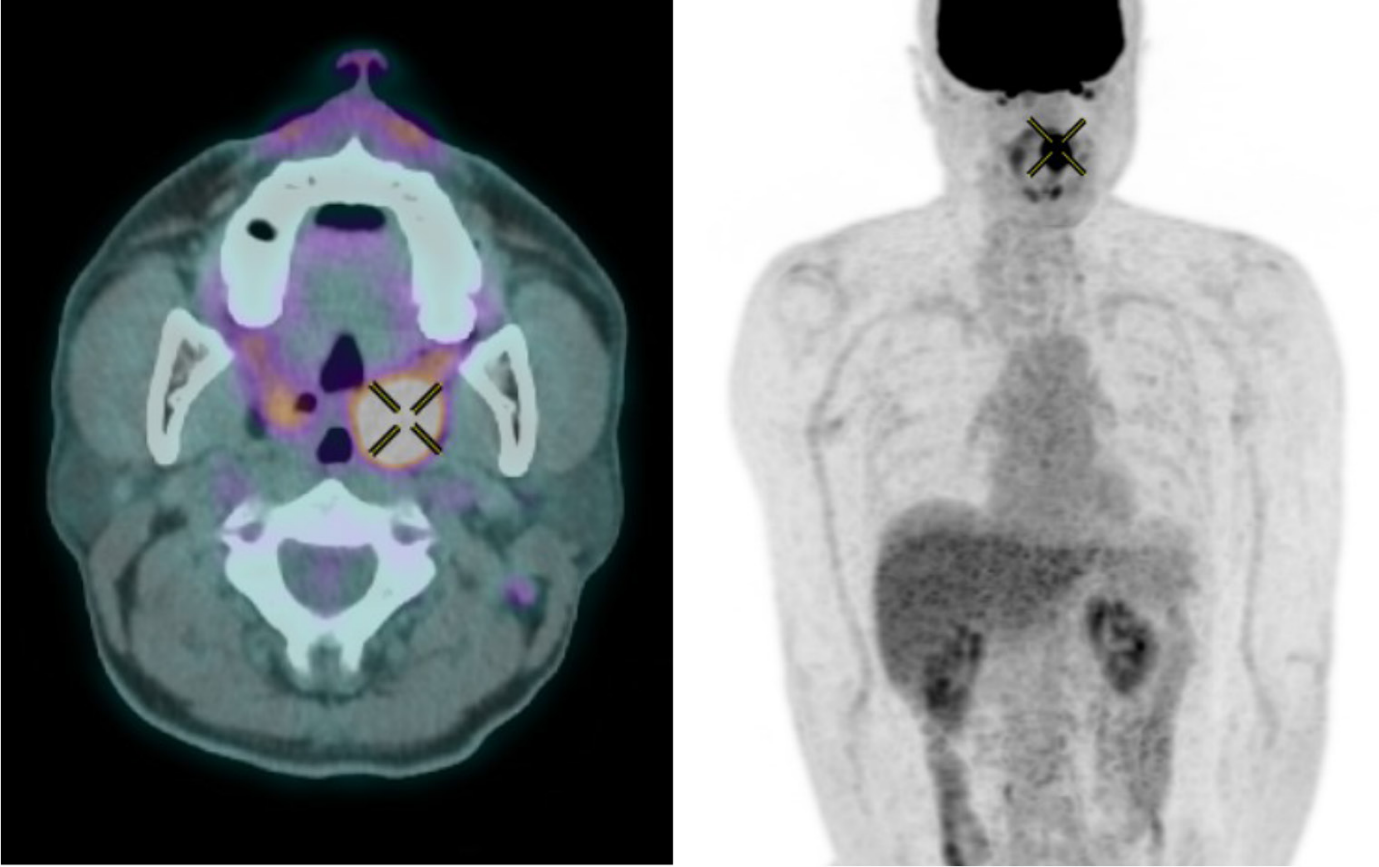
FDG PET showed hypermetabolism in left tonsillar region highly suspicious of malignancy, which proved to be squamous cell carcinoma.
